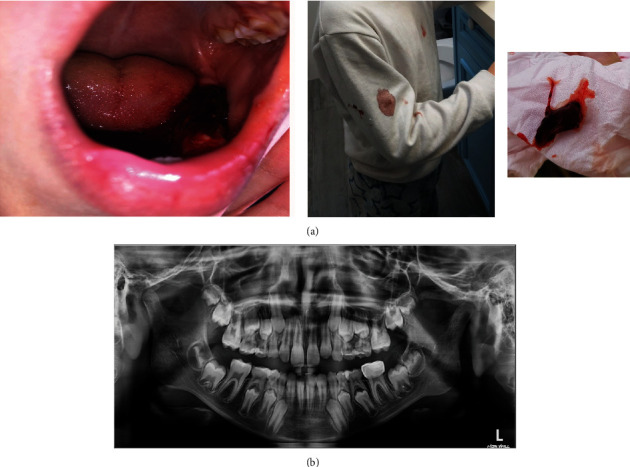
Patient case 3. (a) Bleeding and blood clot 36 hours after placement of SSC on his permanent lower left first molar. (b) Six months postoperatively, no radiographic pathology associated with the SSC on a permanent lower molar.
